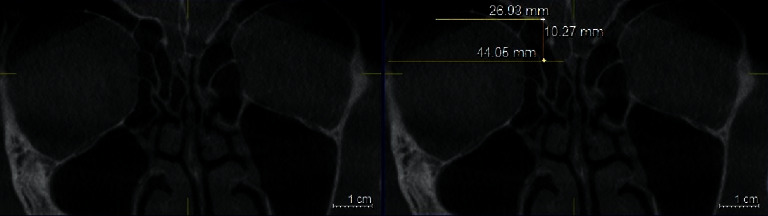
Coronal plan of CBCT demonstrating type 3 Keros classification on the right side.
